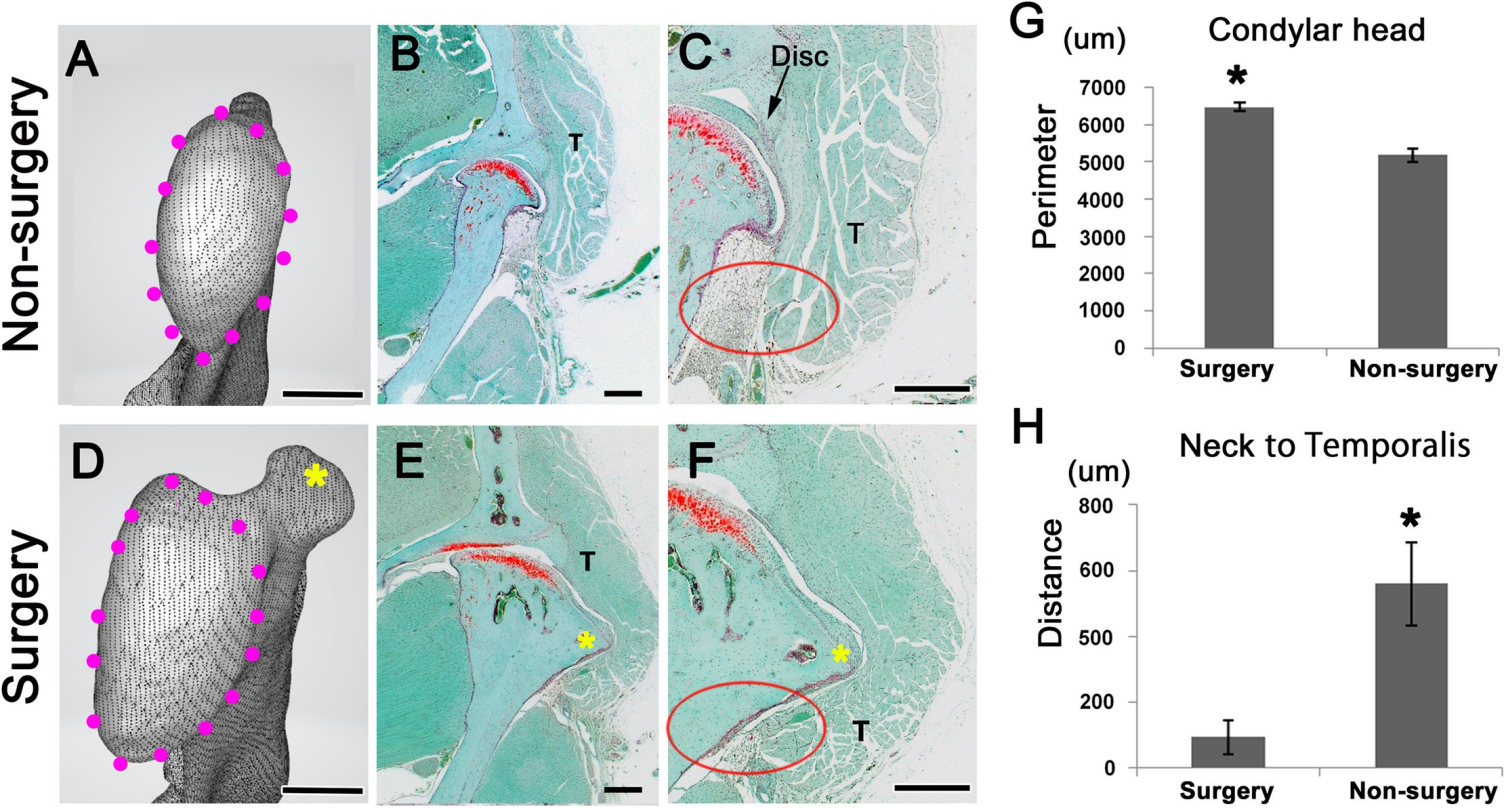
Impact of bone deformation on muscle shape. CT images show the top of the condylar head (panels A and D). Panels B and E show the frontal sections. Panels C and F show high magnification view of panels B and E. At 70 days after discectomy, deformation of the mandibular condyle is evident in the temporomandibular joints (panels A, D, and G). The shape of the temporalis muscle (T) has changed after discectomy (panels B, C, E, and F). The muscle on the discectomy side is not only in contact with the condylar head but is also in contact with the condylar neck (panels F and H; oval). The muscle on the non-surgery side is separated from the condylar neck (panels C and H; oval). Scale bar: 500 μm (panels A-F).
